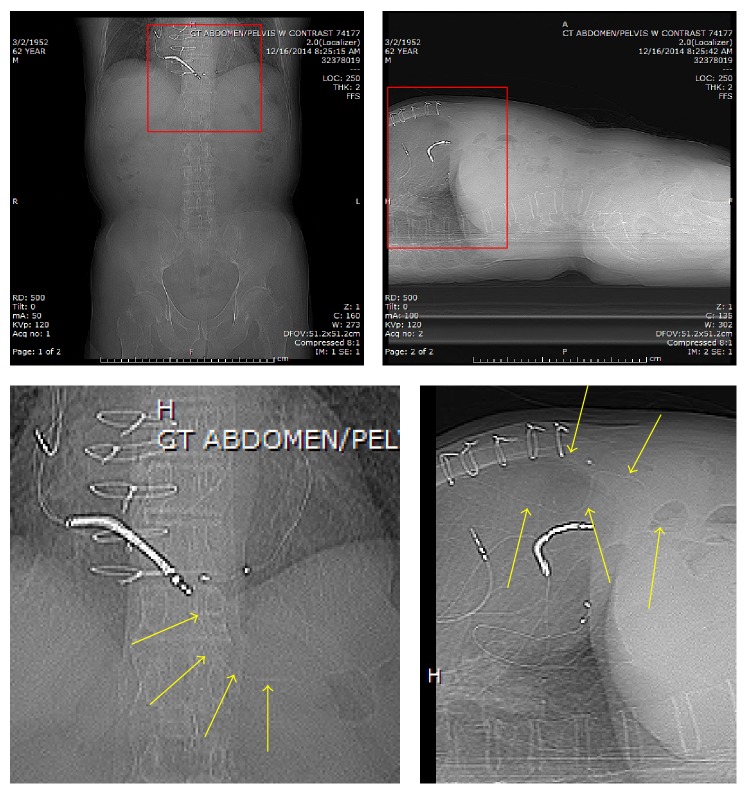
Scout images demonstrating faint linear hyperdensities (yellow arrows) vertically oriented descending below the diaphragm. The lateral scout view demonstrates the distal most aspect of the wires overlying air-filled transverse colon (found to be intraluminal on the following CT).
